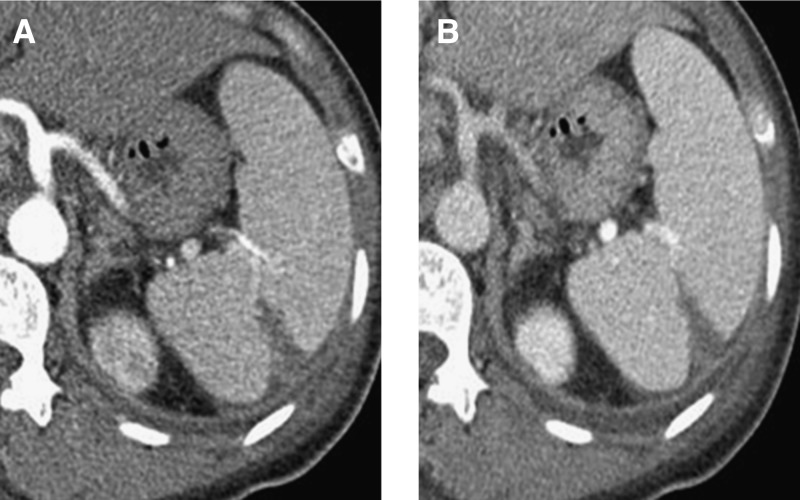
Abdominal computed tomography with enhancement shows a low-attenuated, wedge-shaped lesion consistent with splenic infarction as well as splenomegaly in the arterial phase (A) and portal phase (B).
